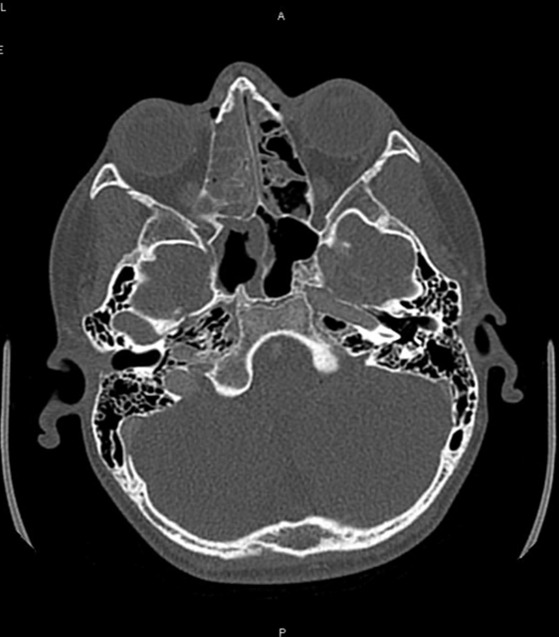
Computed tomography of our patient showing right ethmoid (white arrow) and maxillary (white arrowhead) sinusitis with enhancement demonstrating abscess formation (arrow).
